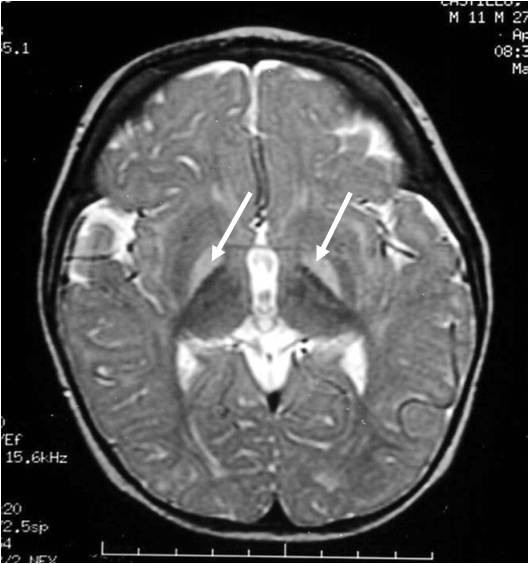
Magnetic resonance imaging of the head. Hyperintense basal ganglia lesions on T2-weighted images.
